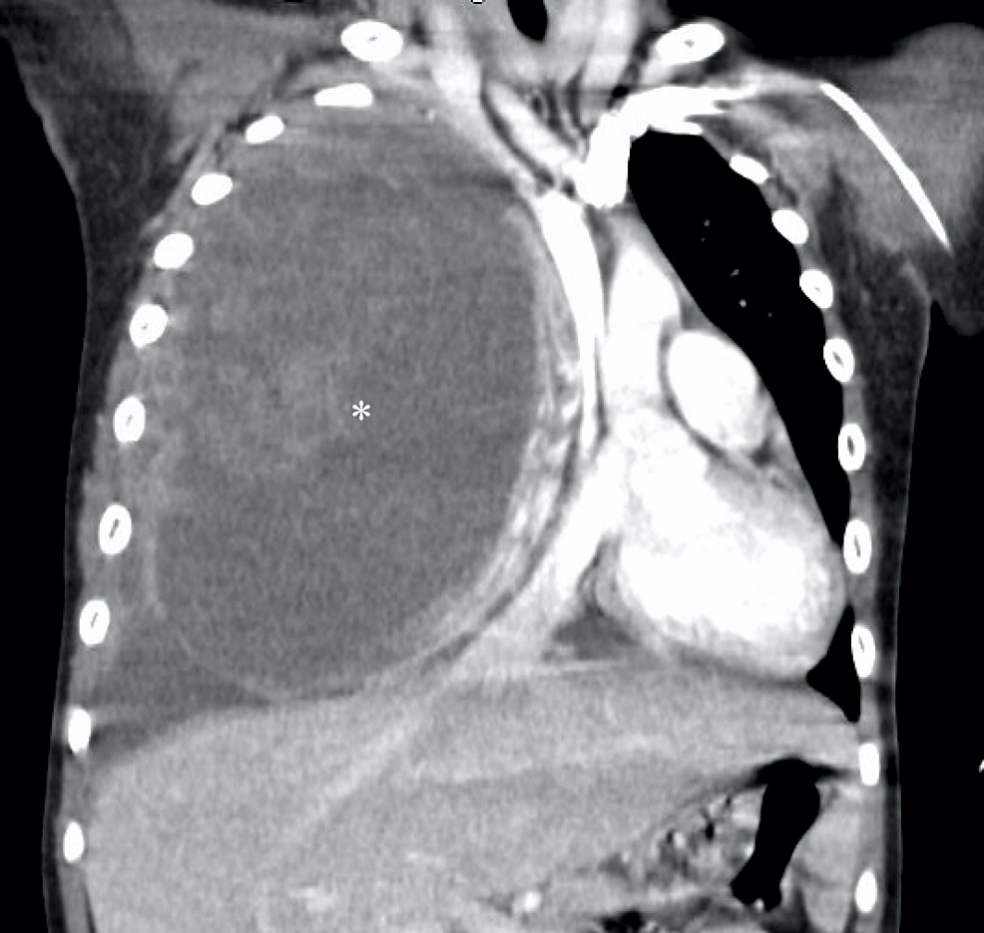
CT scan showing a large mass occupying almost the entire right thoracic cavity with mediastinal displacement Mass measures approximately 13 cm AP by 10 cm transverse by 15 cm craniocaudal. There is evidence of invasion of the lateral right sixth rib and chest wall. There is a near-complete collapse of the right lung with a mediastinal shift to the left.
